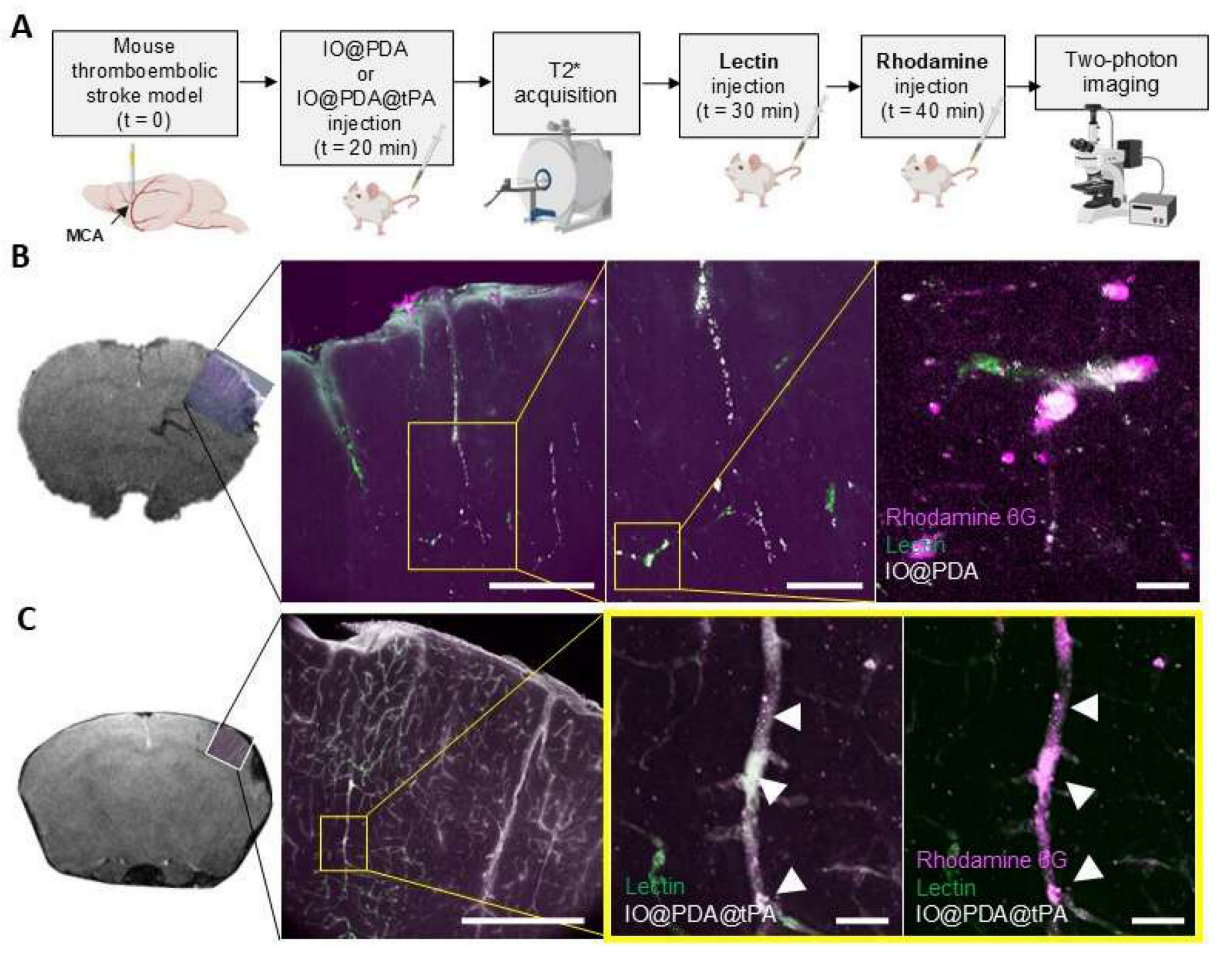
IO@PDA and IO@PDA@tPA are effectively localized in microthrombi. Experimental design (A). MRI acquisition and corresponding IHC: IO@PDA in white, microthrombi in magenta (Rhodamine 6G) and vessels in green (lectin) (B). MRI acquisition and corresponding IHC: IO@PDA@tPA in white, microthrombi in magenta (Rhodamine 6G) and vessels in green (lectin) (C). Scale bar = 20 µm.
